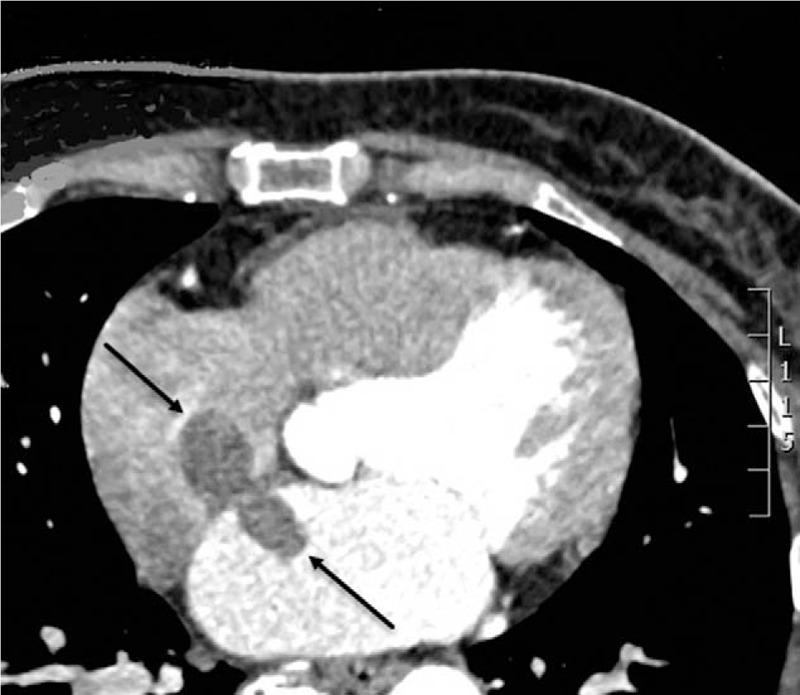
A. computed tomography scan showing a mass in both atria. The black arrow shows the myxoma in the right atrium and the left atrium.
